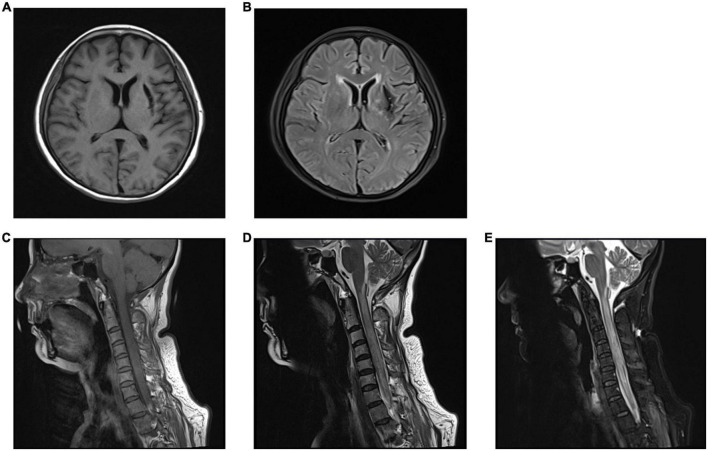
The brain and cervical spine magnetic resonance imaging (MRI) performed at 2 weeks. (A,B) Brain MRI showed the left basal ganglia-insular softening foci in T1 (A) and T2 (B) weighted image. (C–E) Cervical spine MRI showed diffuse hyperintense signal in the cervical cord in T2-weighted image (D) and fluid-attenuated inversion recovery (FLAIR) images (E), and normal findings were observed in T1-weighted image (C).
